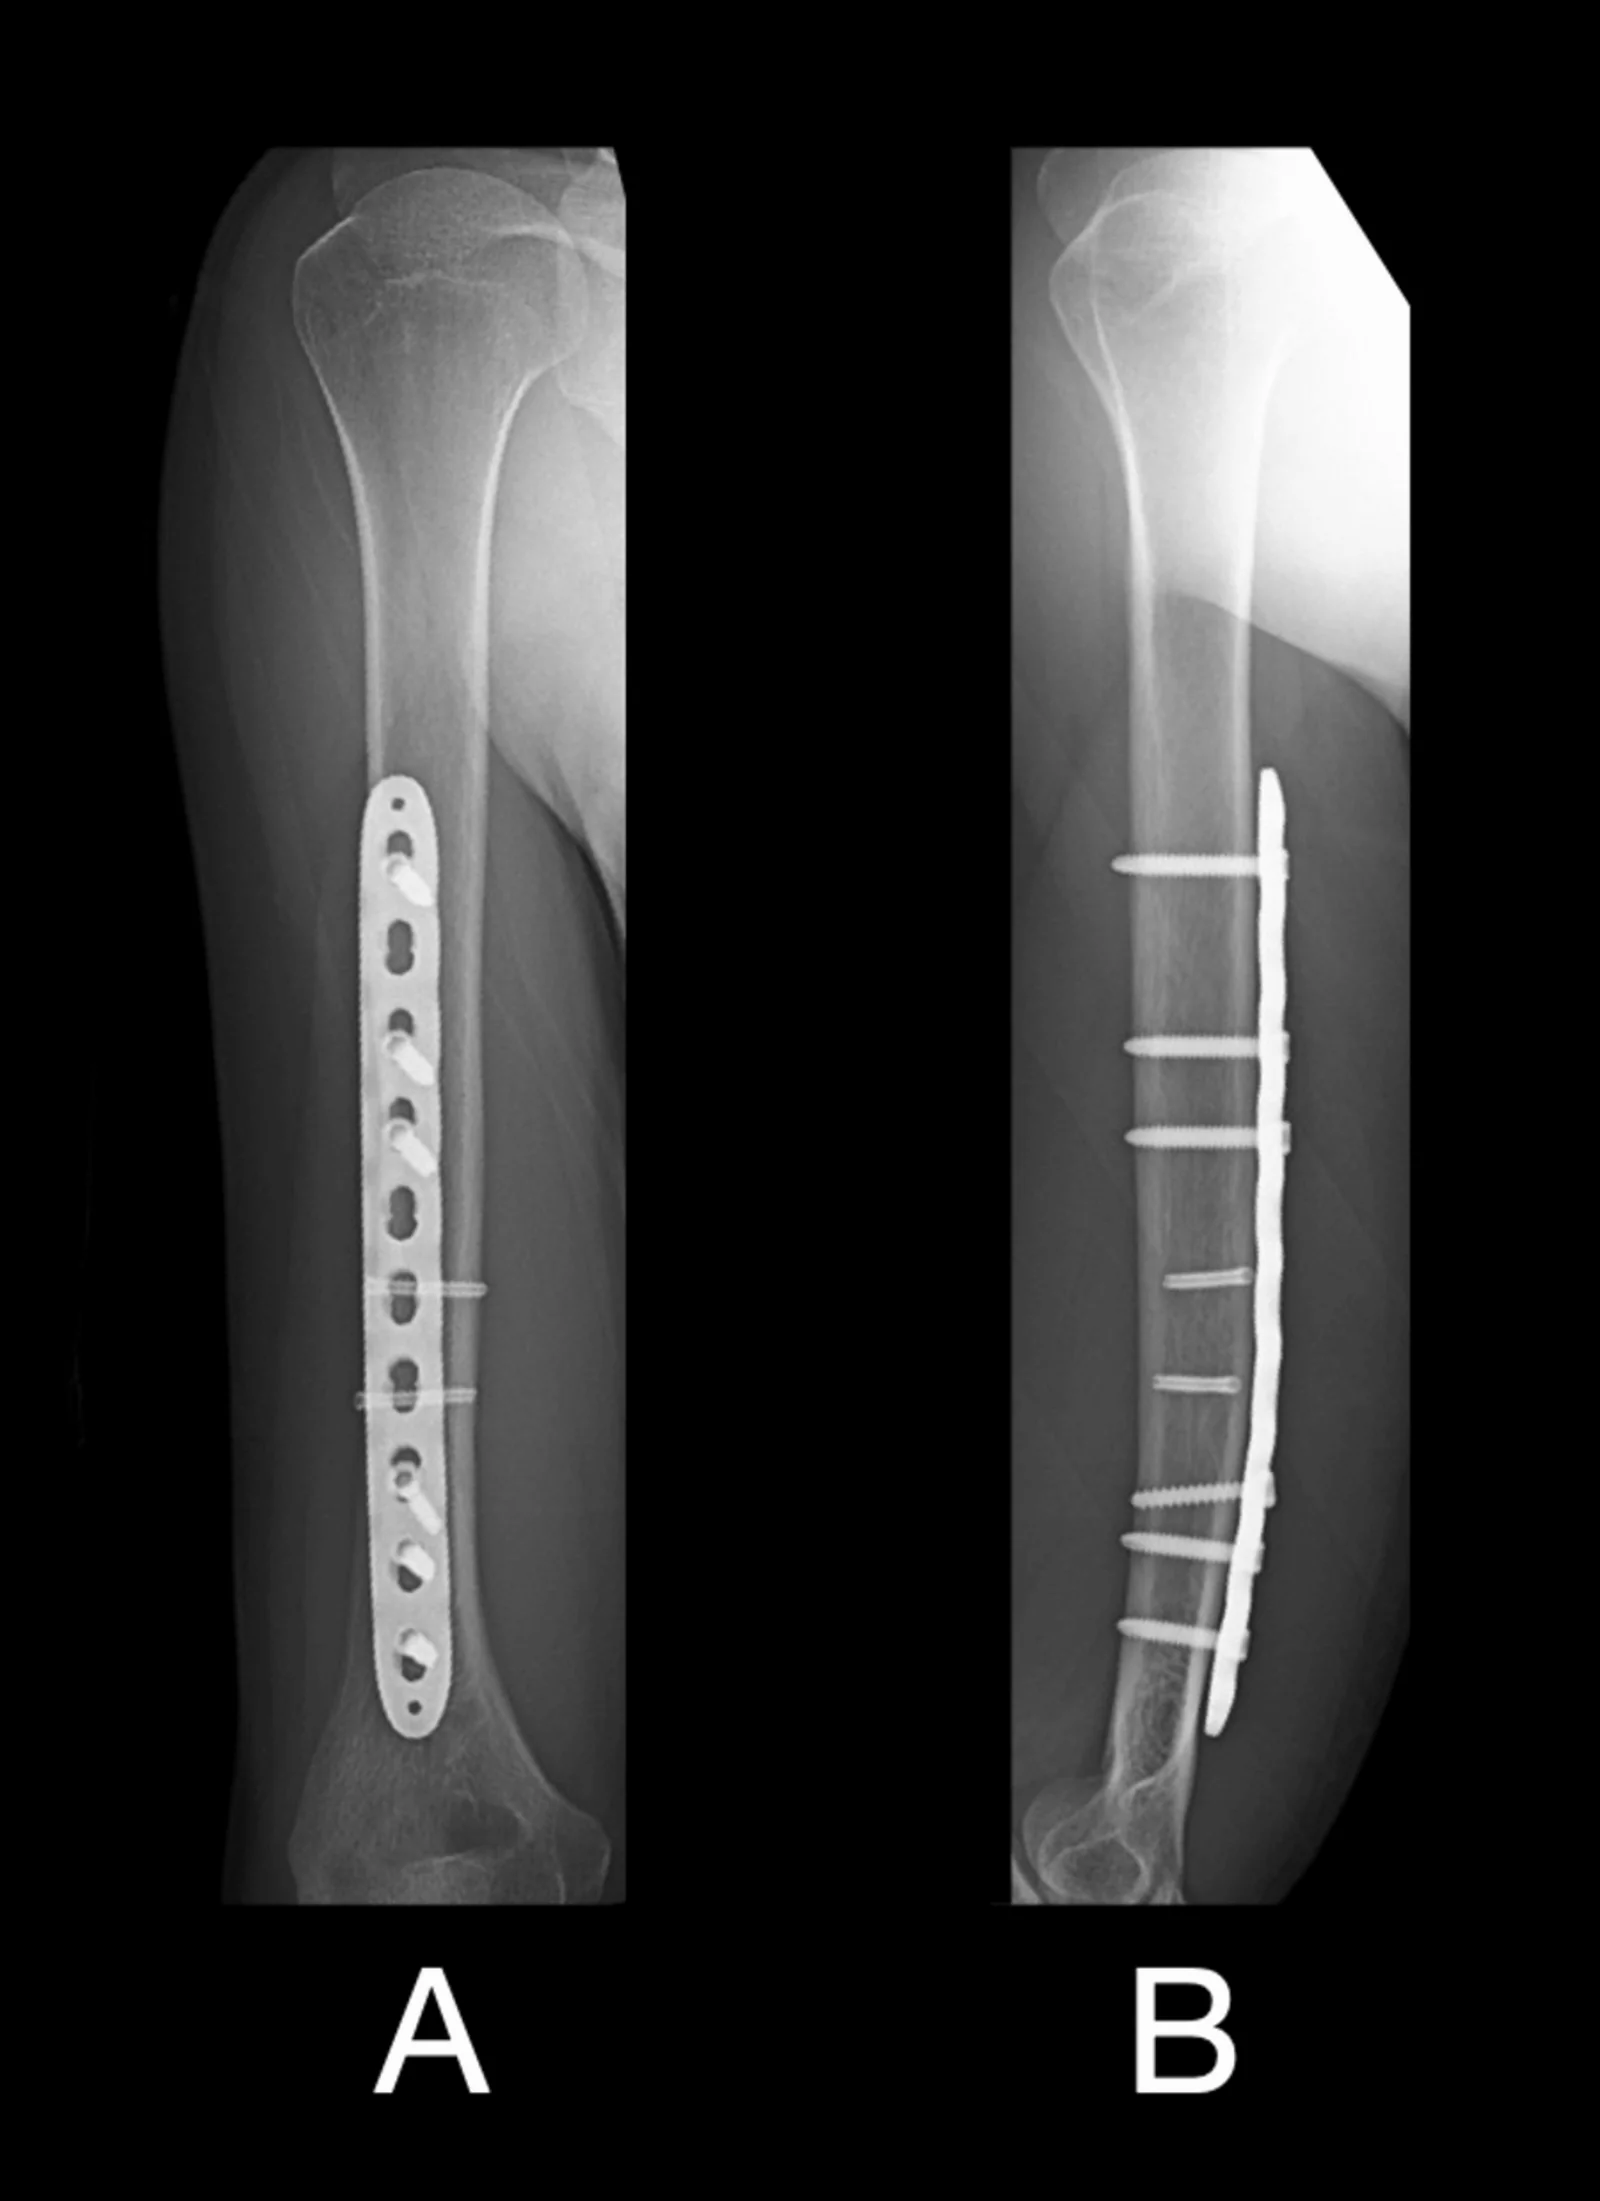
Postoperative radiographs after posterior plate fixation. Postoperative anteroposterior and lateral radiographs demonstrating posterior plate fixation with satisfactory fracture reduction and implant position.
